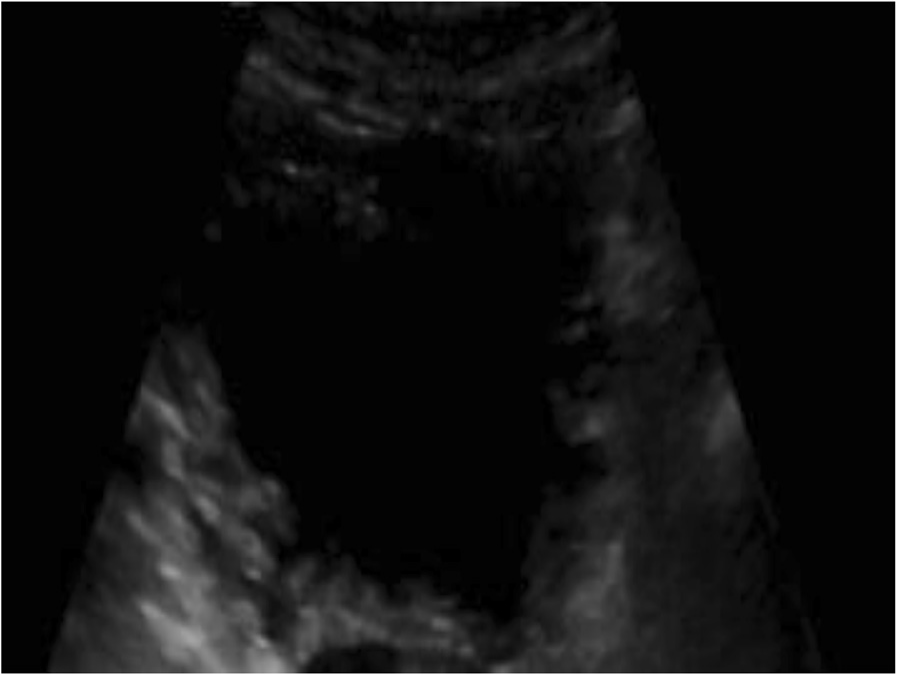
Bladder ultrasound showing trabeculation and bladder wall thickening.
